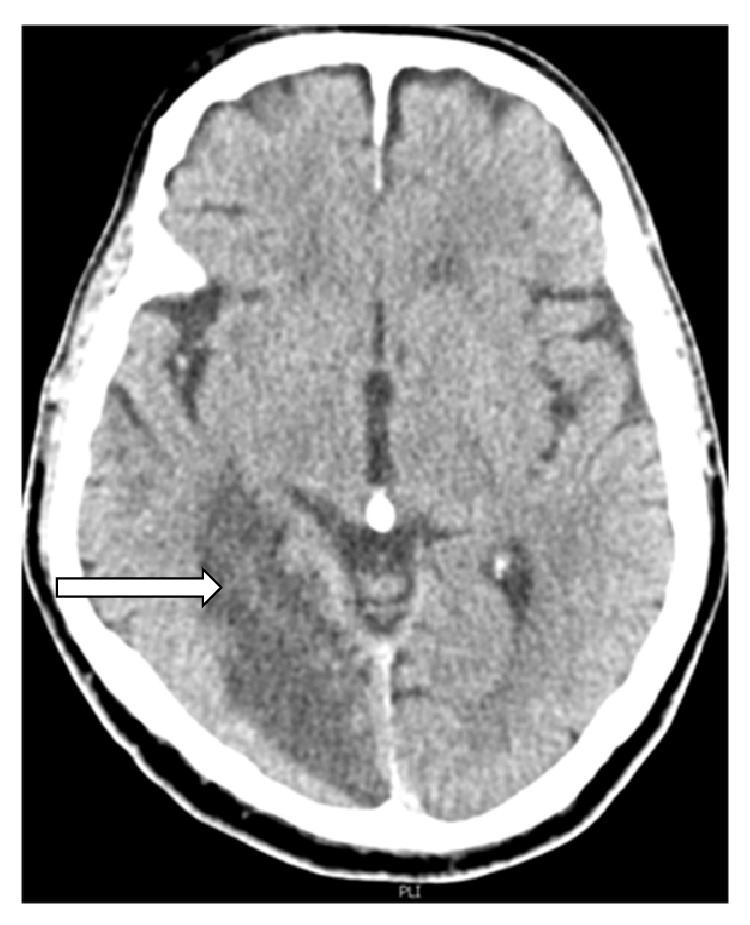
An axial CT image of the brain shows classic nonhemorrhagic cerebral infarction in the right posterior cerebral artery territory (*white arrow*).
